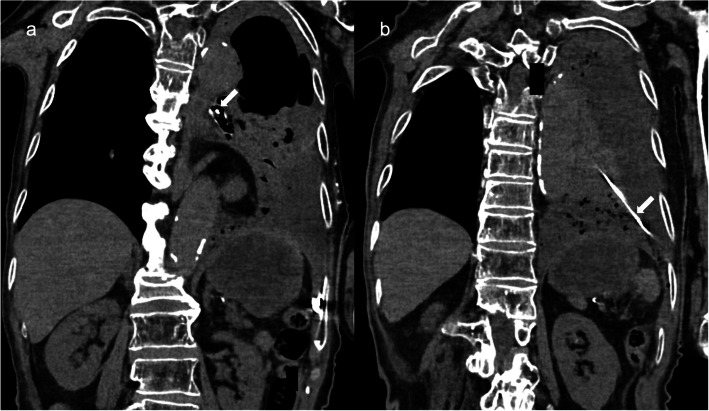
Chest CT images. The nasogastric tube passed through (a) the left main bronchus and (b) into the pleural cavity (arrows)
